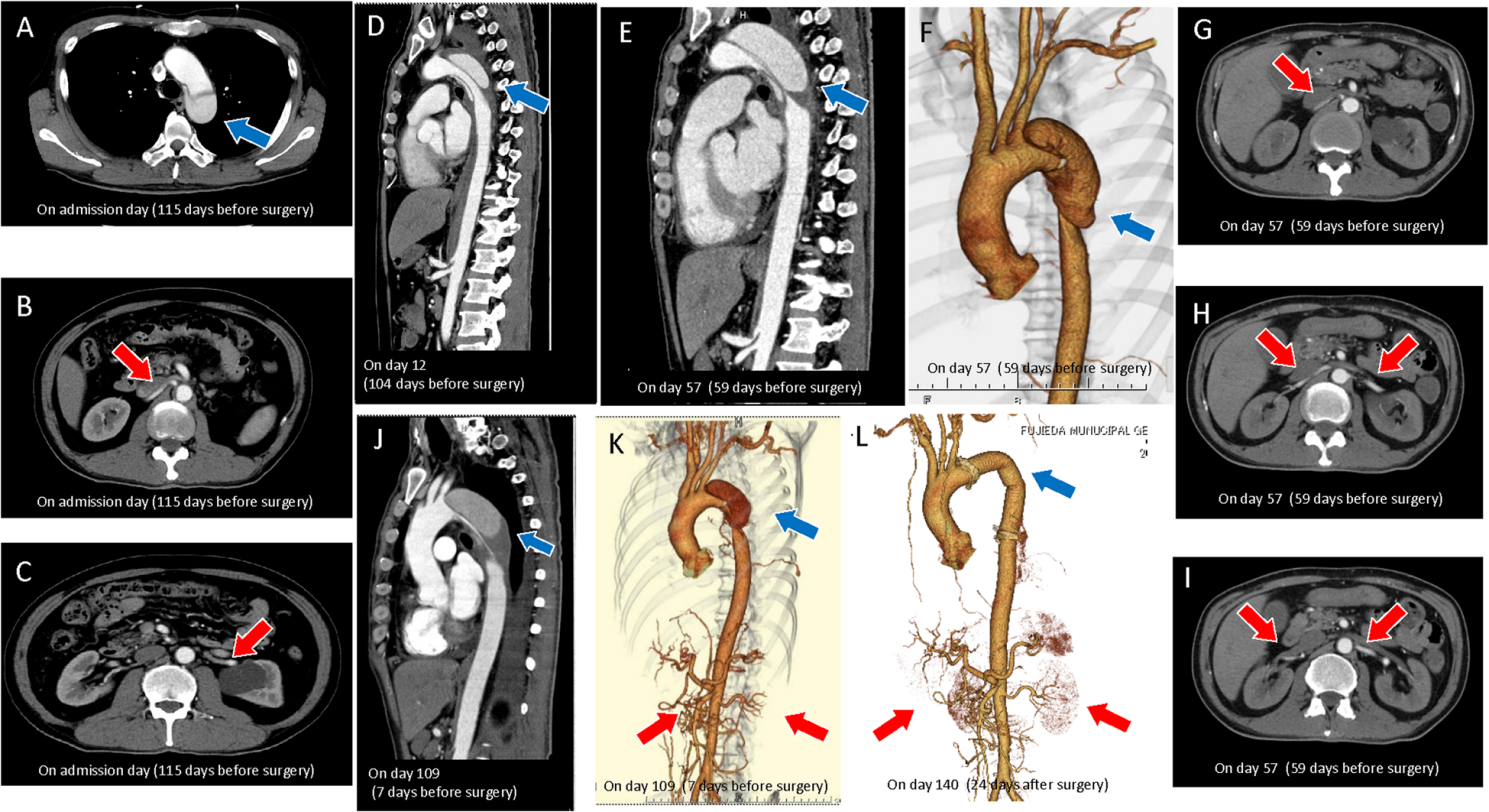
Representative aortic dissection findings upon admission and on hospital days 12, 57, 109, and 140. a-c Two-dimensional, contrast-enhanced computed tomography upon admission shows a Stanford type B aortic dissection originating from the left subclavian artery (a) to the abdominal aorta at the level of the renal arteries (b). An axial section shows no aortic dissection at the level of the renal arteries and equal contrast enhancement in both kidneys (c). Sagittal two-dimensional computed tomography (CT) angiography image obtained on day 12 (blue arrow) showing that the true lumen was more severely compressed by the false than that denoted on the scan taken just after admission (d). (e-i) CT scans of day 57. Sagittal view recorded by two-dimensional computed tomography (CT) angiography (e), three-dimensional CT angiography (f), and axial sections (g-i) showing the true lumen more severely compressed by the false lumen (blue arrow) than that noted on the scans taken on day 12. j Sagittal view recorded pre-operatively on two-dimensional CT angiography showing the true lumen more severely compressed by the false lumen (blue arrow) than that noted in the scan taken on day 57. k Pre-operative three-dimensional CT angiography reveals the false lumen tightly compressing the true lumen (blue arrow). Despite the compression on the thoracic part (blue arrow), on the abdominal level there is no anatomical obstruction; no static obstruction of the renal arteries is evident (red arrows). l After aortic graft replacement, three-dimensional CT angiography indicated that the patency of the aorta was successfully re-established (blue arrow). Changes in the morphology of the renal arteries are not apparent (red arrows). Blue arrows indicate the site of existing dissection (a, d-f, j, k), and the site where the graft was inserted (l); red arrows indicate renal arteries (b, c, g-i, k, l)
